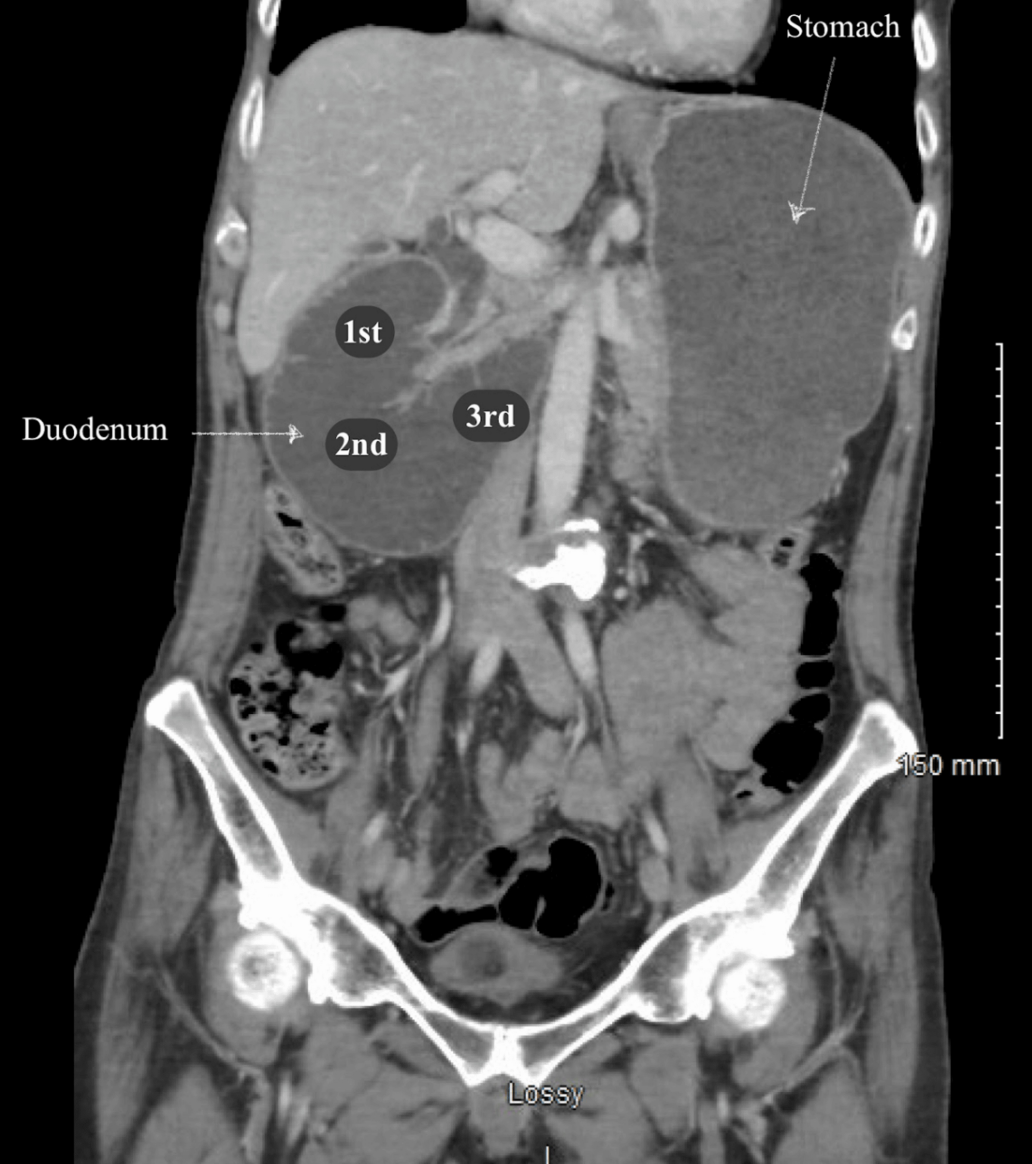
Coronal computed tomography (CT) scan displaying the compression part of the duodenum between the SMA and aorta In this image, the labeled numbers represent the portions of the duodenum. This CT scan highlights compression toward the third portion, indicating characteristic features of superior mesenteric artery (SMA) syndrome. In addition to the compression, the stomach appears to be dilated, possibly due to the obstruction of the gastric outlet.
